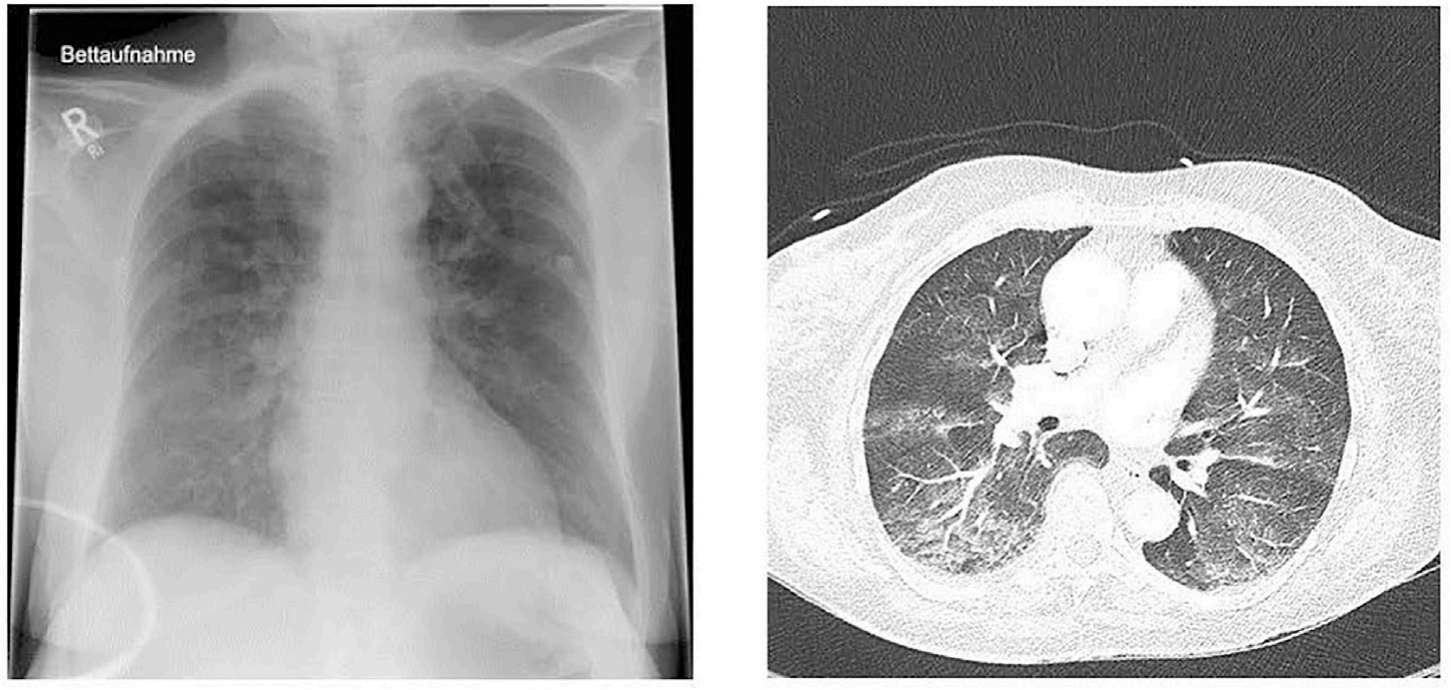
Chest x-ray (left) and computed tomography (right) of a 72-year-old previously healthy and physically fit female after swimming in a cold lake (Courtesy of Karin Hasmiller, MD, Berufsgenossenschaftliche Unfallklinik, Murnau, Germany).
